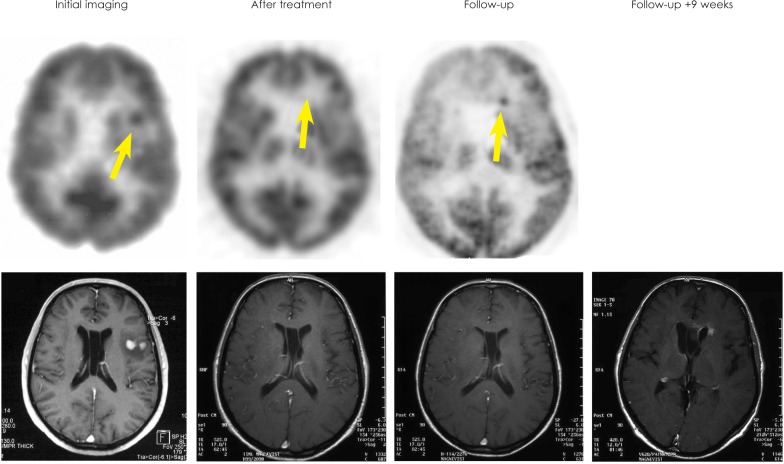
Baseline PET and MRI of patient No. 2 shows left temporal lesion. No pathological FDG-uptake was observed after therapy but a new lesion in the anterior horn was seen on PET 69 weeks later (SUV=10.5). MRI at this time point was still categorized as complete remission. A contrast-enhancing lesion appeared on MRI 9 weeks thereafter.
